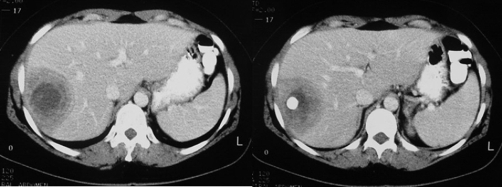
Contrast-enhanced CT scan reveals a hypoattenuating lesion in the right hepatic lobe with peripheral enhanced thickened wall. A central dense calcium deposit is also observed.
